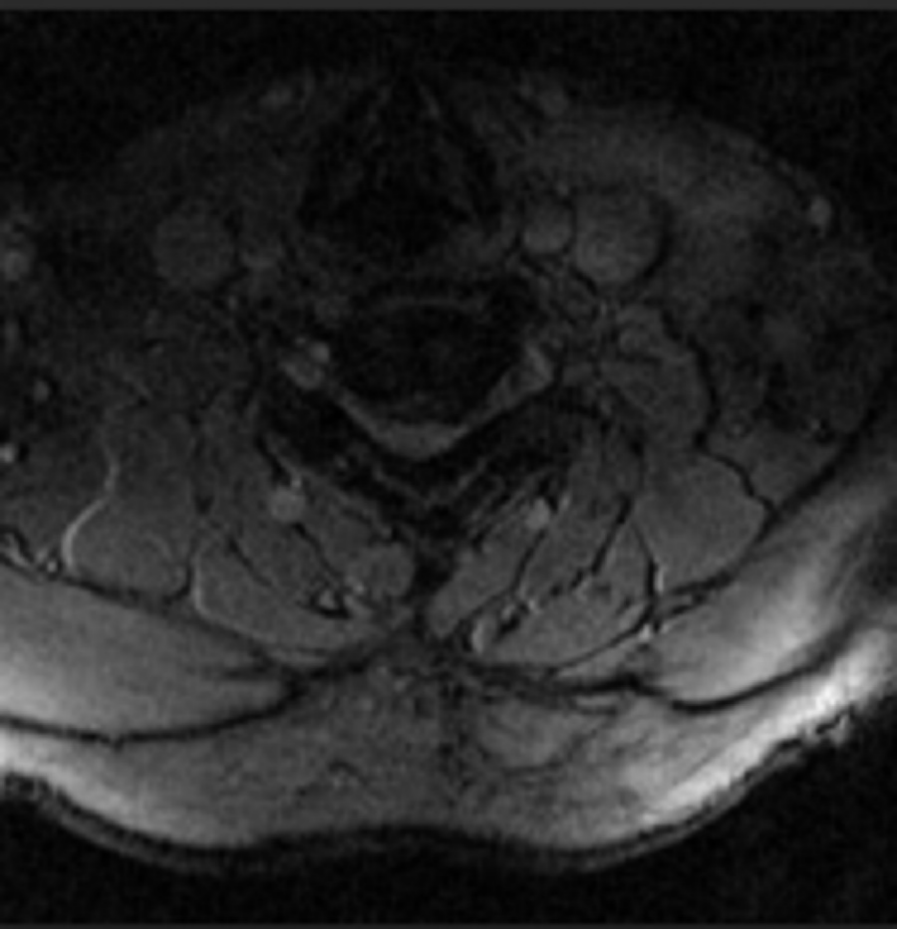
(Case 1) Preoperative axial MRI of cervical spine at C5/6
